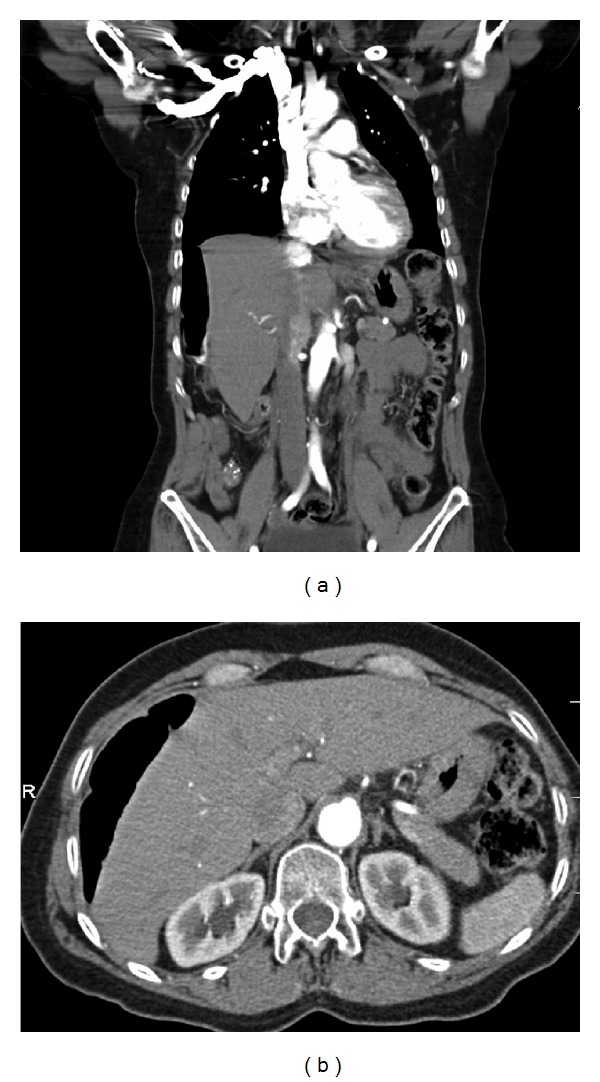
(a) Coronal CT view. (b) Axial CT view. Both demonstrate a loop of colon interpositioned between the liver and right hemidiaphragm.
